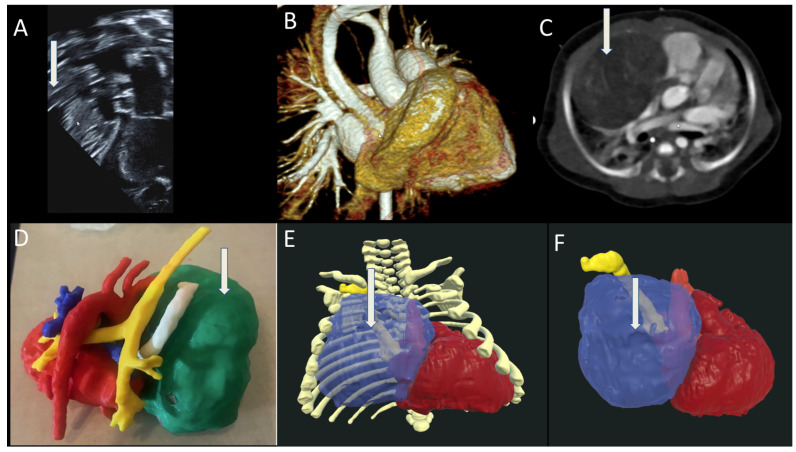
(A) TTE 2D showing teratoma on the right side of the chest, arrow; (B,C) chest CT revealing the teratoma in the right chest with close proximity to the SVC and the ascending aorta, leftward displacement of the heart; (D) 3D print; (E,F) VR segments of the teratoma in blue, SVC in yellow, heart in red.
